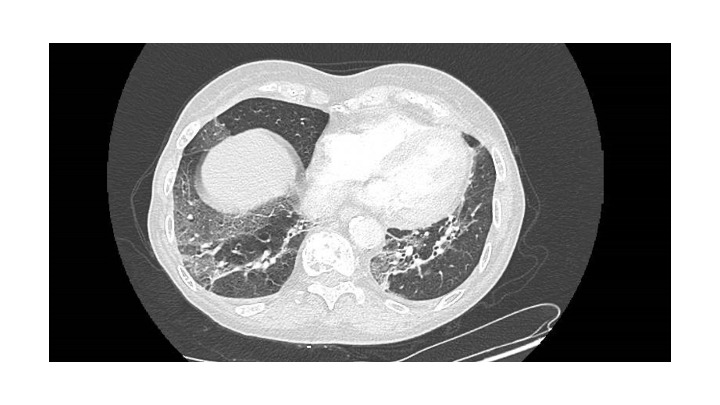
Thoracic CT-scan, showing SARS-CoV-2 pneumonia with severe involvement, affecting between 25 and 50% of the lung parenchyma, with associated atelectasis bands
